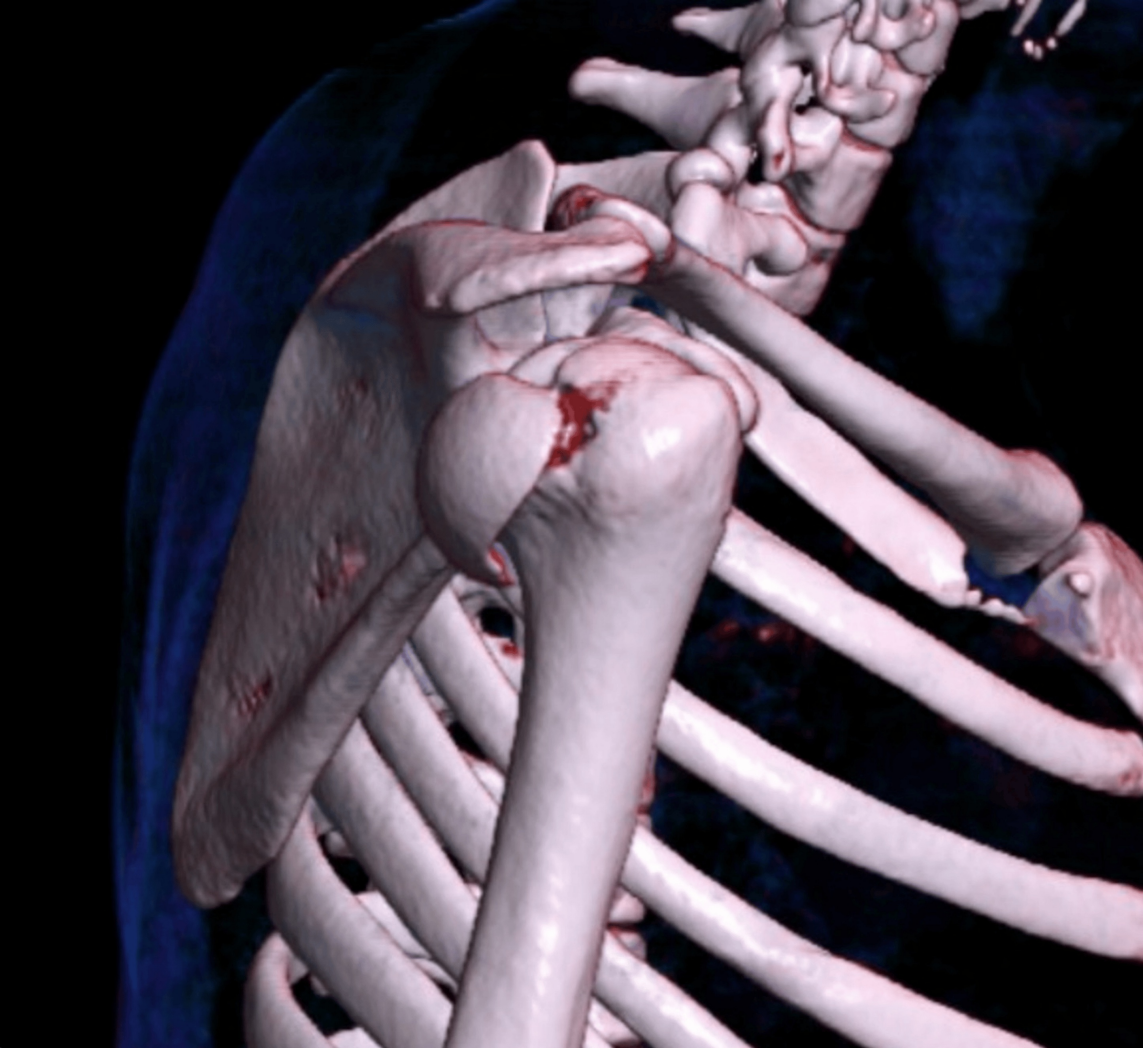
Initial 3D CT reconstruction
